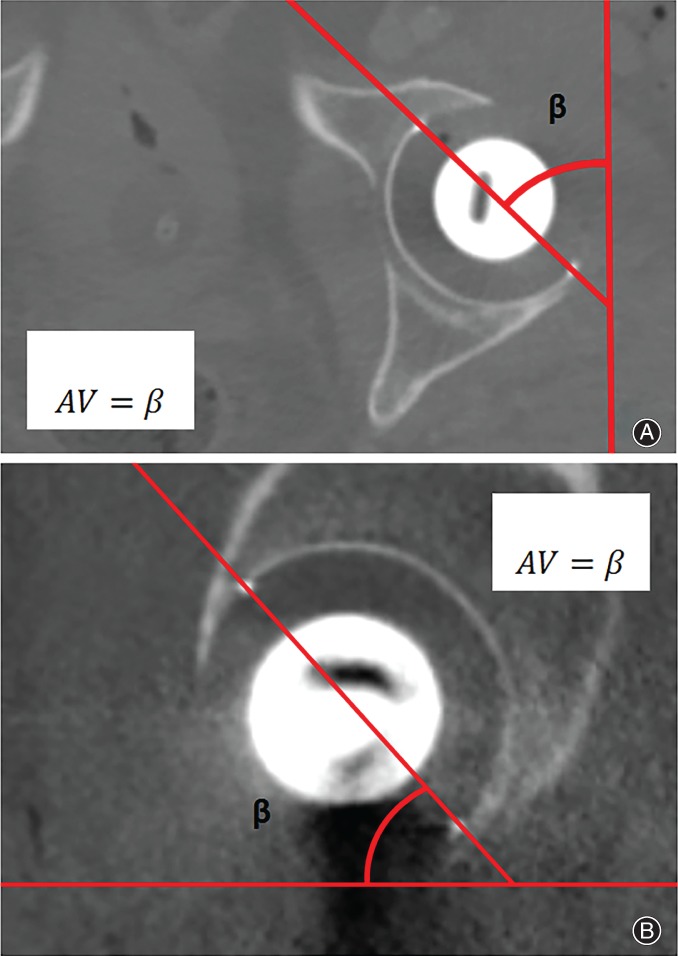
Category 1 methods. Definitions and algorithms of the included anteversion (AV) measurements methods with CT. The angle is measured by the opening of the cup in relation to the axis of the respective plane. (A) Transverse‐CT anteversion. (B) Sagittal‐CT anteversion.
